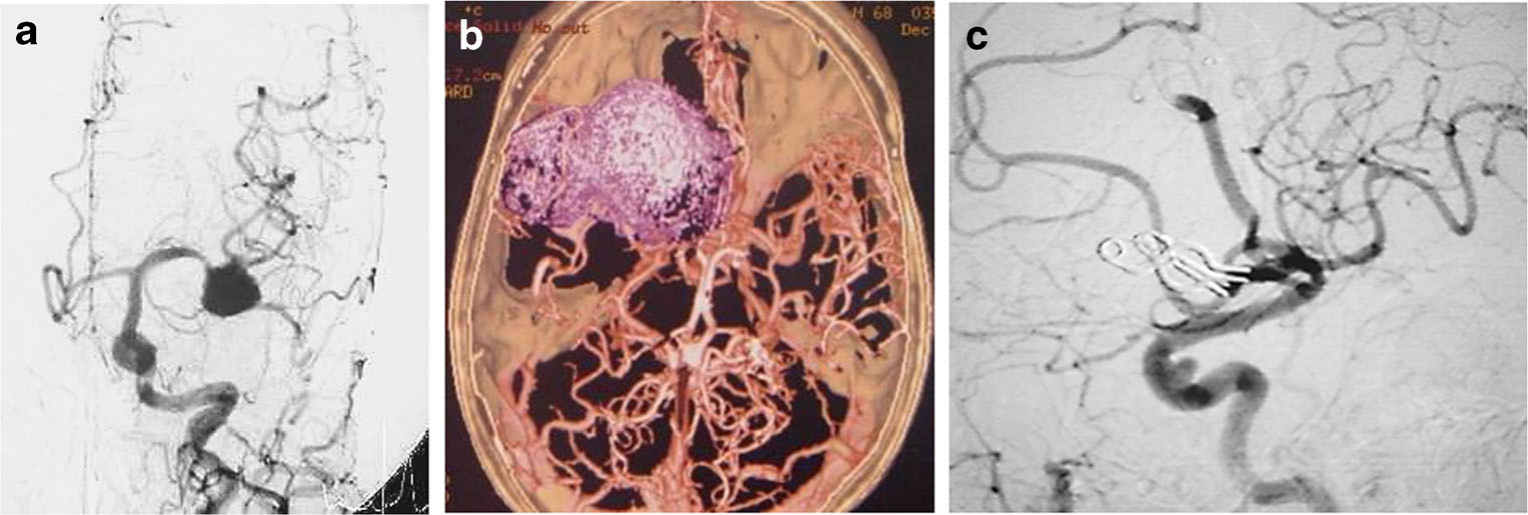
A woman with a giant thrombosed MCA aneurysm. She suffered a subarachnoid hemorrhage. a Left common carotid angiogram showed an irregularly shaped aneurysm of the left MCA. b The three-dimensional CT angiogram reveals the space occupied by the aneurysm. c Postoperative angiogram of lateral view showing complete disappearance of the MCA aneurysm after multi-clip occlusion, and intact M1, M2, and the flow from STA to M2 via short saphenous vein graft
